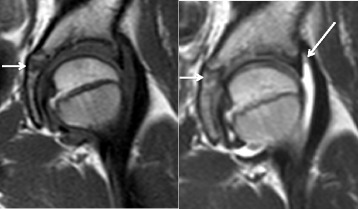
Patient magnetic resonance images. Coronal T1 (*left*) and T2 magnetic resonance images (*right*) showing that the mass was characterized by low intensity on T1 images and high intensity on T2 images (*arrows*). Magnetic resonance imaging also showed joint effusion (*long arrow*). Together with clinical findings and computed tomography images, the small lesion was diagnosed as osteoid osteoma of the acetabulum
